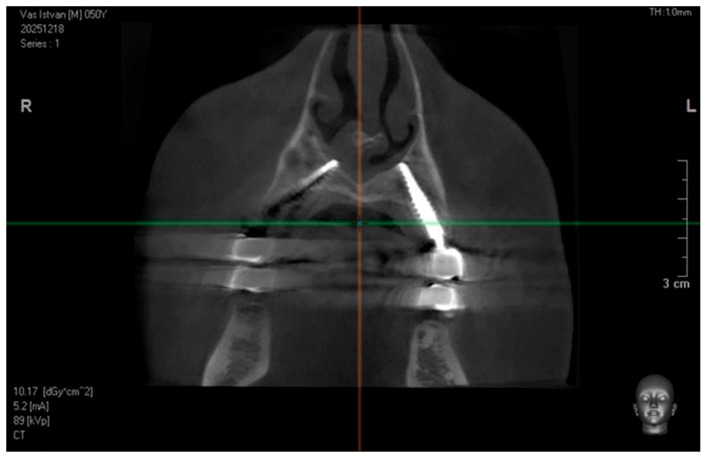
Postoperative coronal CBCT image demonstrating bilateral implant positioning and the achieved anchorage pattern, confirming stable placement and appropriate angulation in relation to surrounding anatomical structures.
